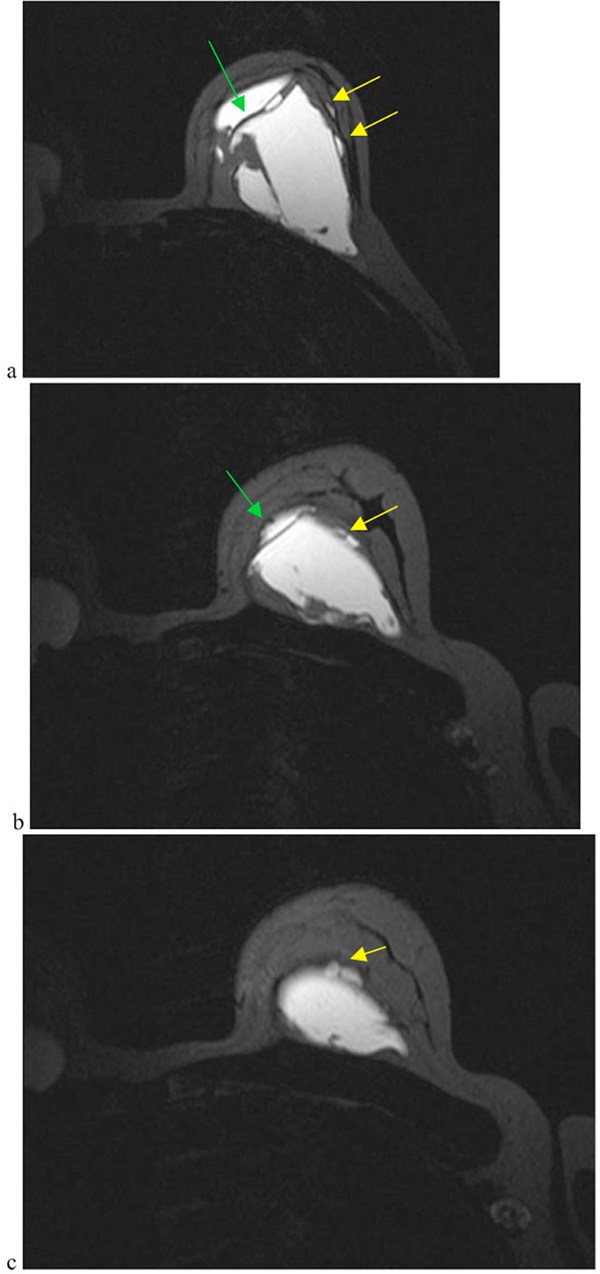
Magnetic resonance imaging of the breasts. Axial T1 weighted fat suppressed images through the left implant (a)–(c) demonstrate extracapsular silicone (yellow arrow) with gross disorganisation and collapse of the implant with a positive "linguine sign" (green arrow). Features are of a collapsed intra and extracapsular rupture.
